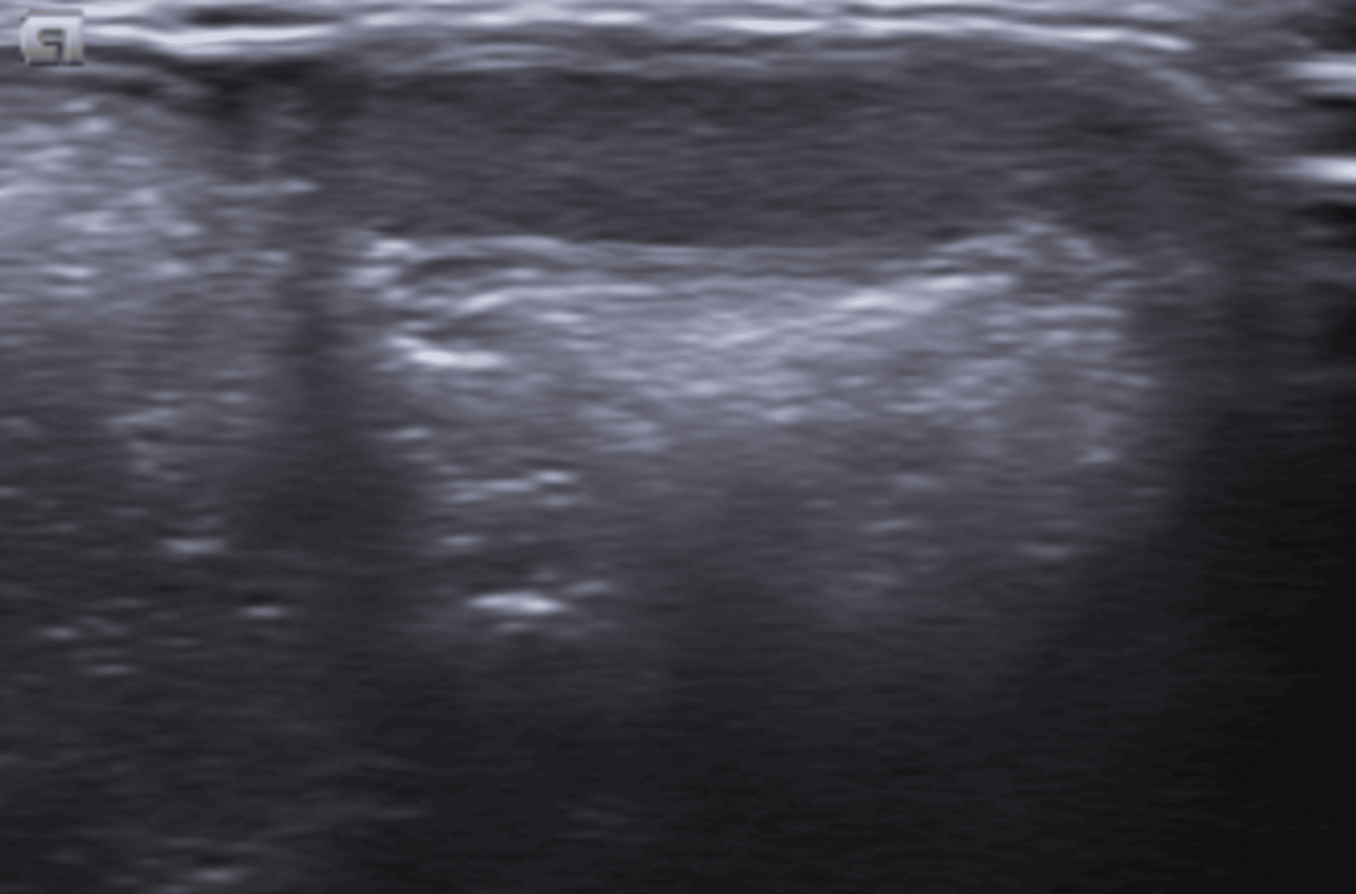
Grayscale abdominal wall ultrasound A defect in the abdominal wall is identified in the left lower quadrant, with herniation of a sac containing an ovoid structure compatible with a testis. The testis demonstrates decreased echogenicity and size compared to expected, suggesting testicular atrophy
